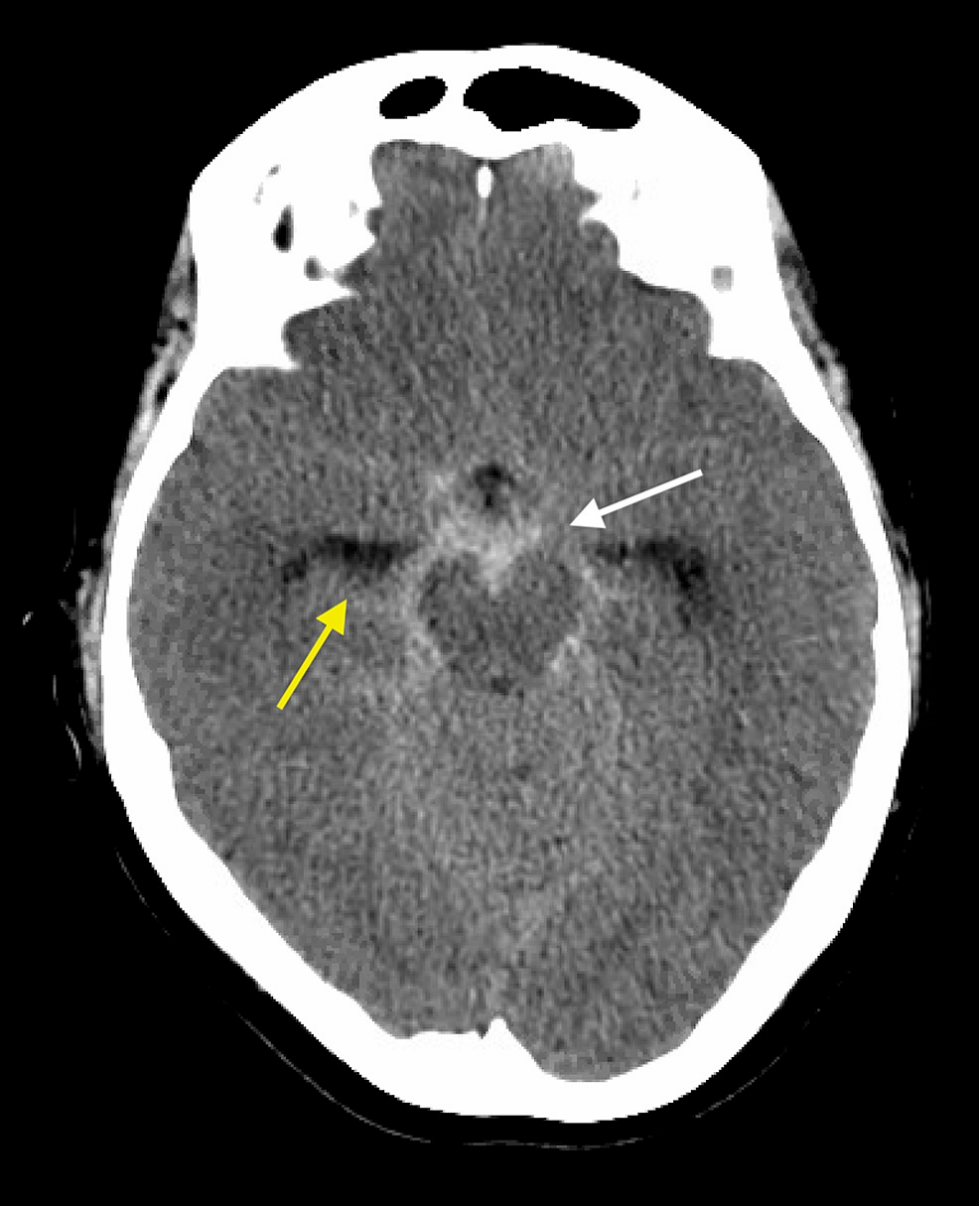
Axial non-contrasted CT head demonstrating diffuse subarachnoid hemorrhage throughout the basilar cisterns (white arrow) and enlargement of the ventricular temporal horns (yellow arrow). CT, computed tomography.
